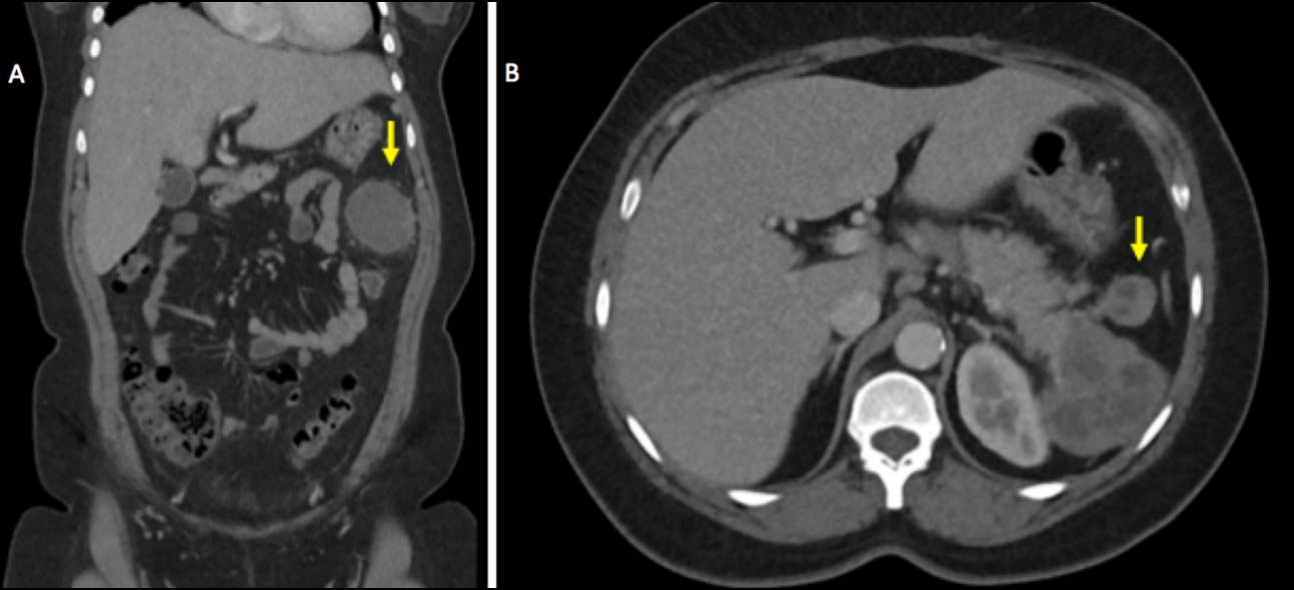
CT A/P imaging showing a heterogeneous mass (A) arising from the pancreatic tail with associated suspected lymph node (B, arrow).
